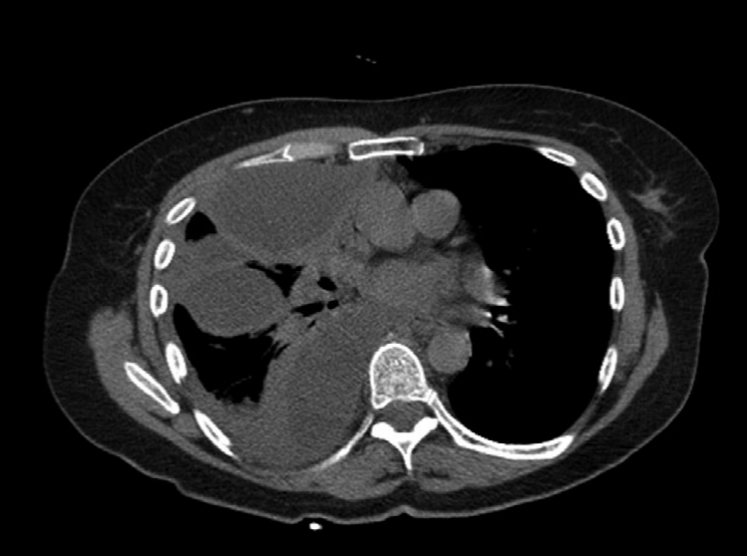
Chest CT scan. Chest CT scan of the patient showing massive right side pleural effusion with atelctasis of the lung.
